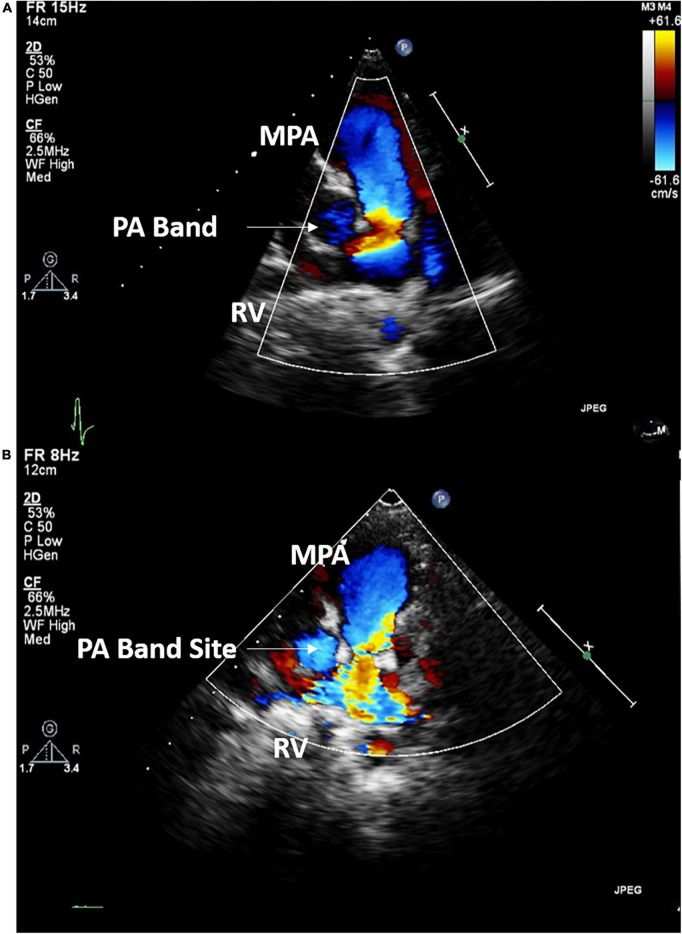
Echocardiographic imaging of Case I before (A) the debanding procedure and at 3-year follow up following the catheter-based debanding (B). RV, right ventricle; MPA, Main pulmonary artery; PA, Pulmonary artery.
